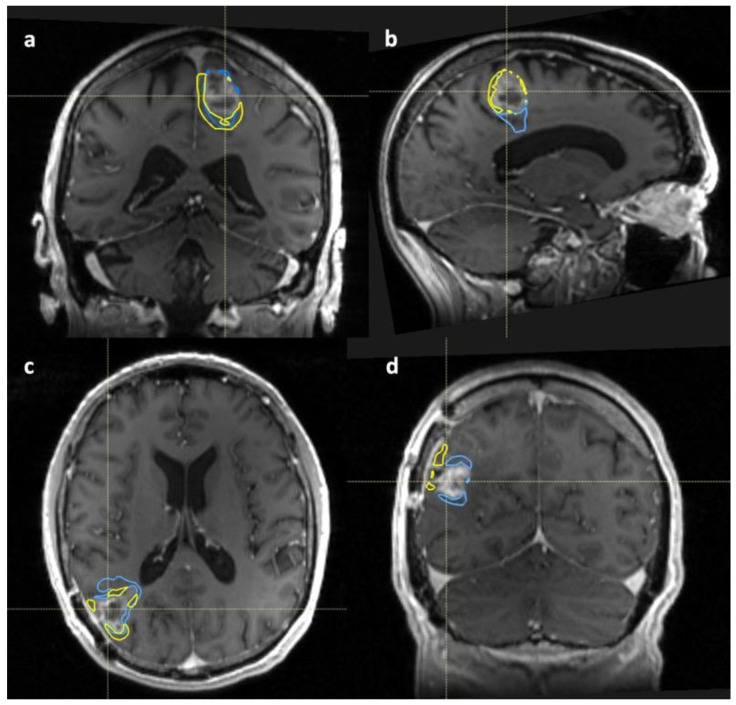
Selected Gd-T1 images from different study timepoints with subtracted DCE-MRI and PET volumes overlaid for GBM 1 (top row) and GBM 5 (bottom row). Volumes are subtracted from the Gd-T1 volume (not shown): Yellow—PET (3 × SUV_max_) volume; Blue—K^trans^ volume. Top row—GBM 1; (a)—pre-RT and (b)—mid-RT images. Bottom row—GBM 5; (c)—pre-RT and (d)—post-RT images.
